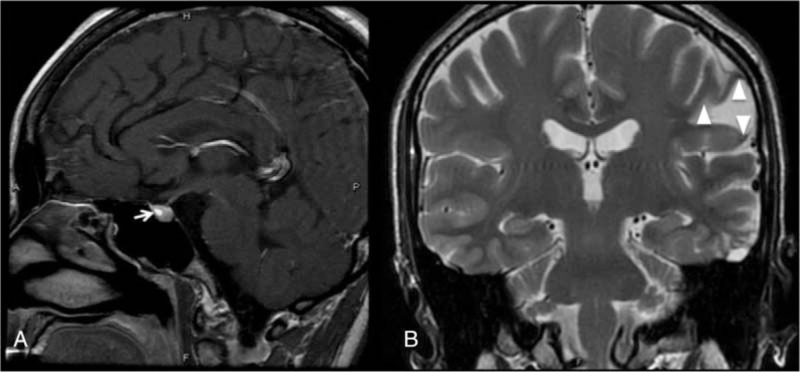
The pituitary microadenoma (arrow) is shown in a sagittal T1-weighted gadolinium contrast-enhanced MRI (A); A coronal, T2-weighted brain magnetic resonance image depicts focal encephalomalacia in the left frontal lobe (arrow head) (B). MRI = magnetic resonance imaging.
